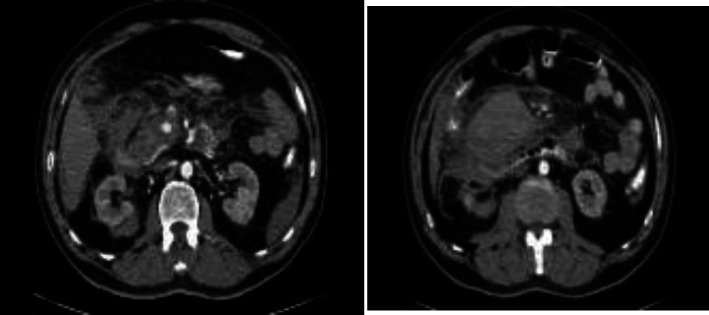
Axial view of Abdominal CT Scan shows Intraperitoneal hematoma
